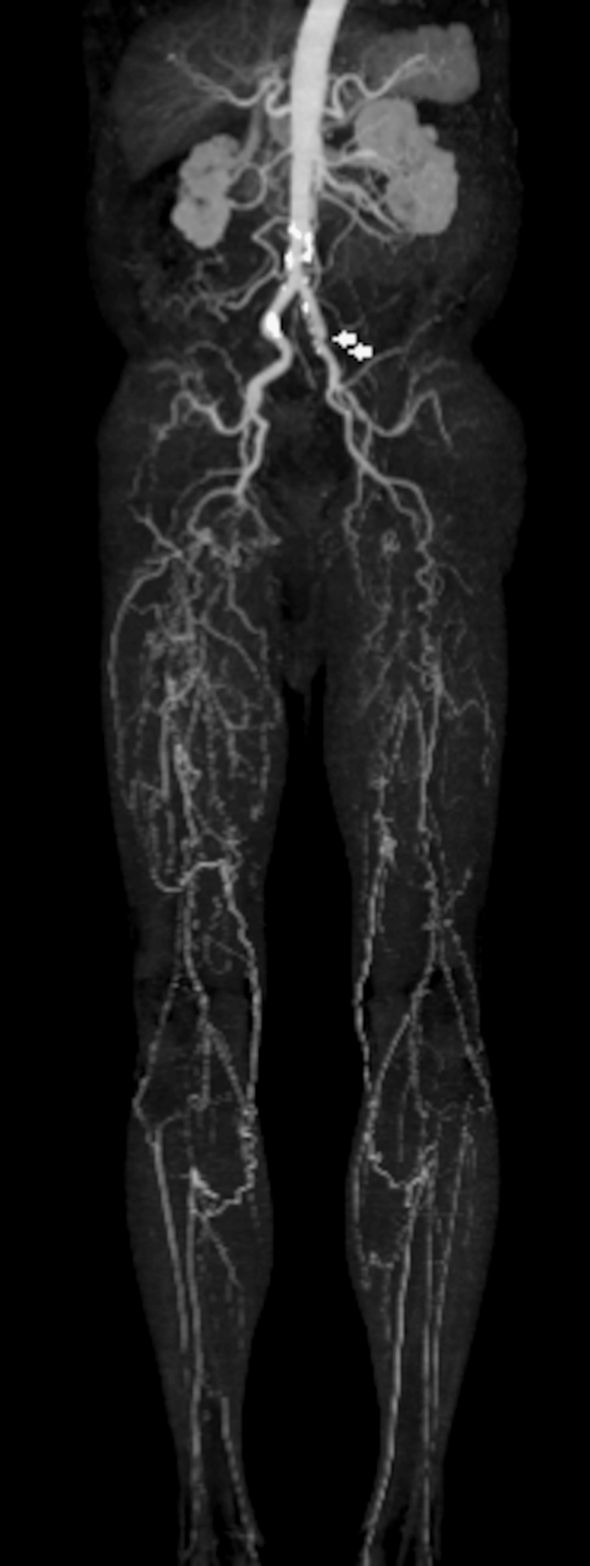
Contrast-enhanced computed tomography image The image is showing exacerbation of IC in the left lower extremity. The left external iliac artery, which was patent at the initial visit, is now occluded at its origin (white arrows).
